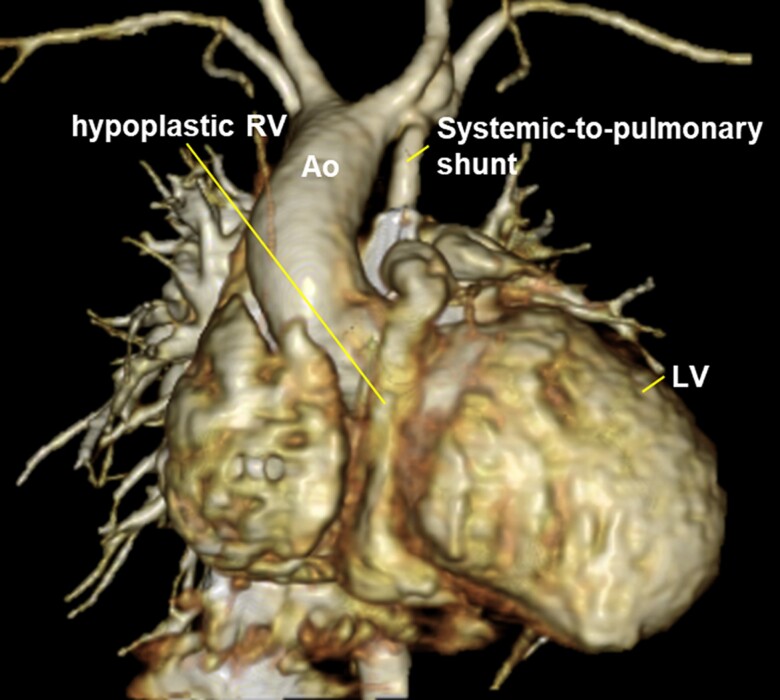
Contrast-enhanced computed tomography showed markedly hypoplastic right ventricle.
